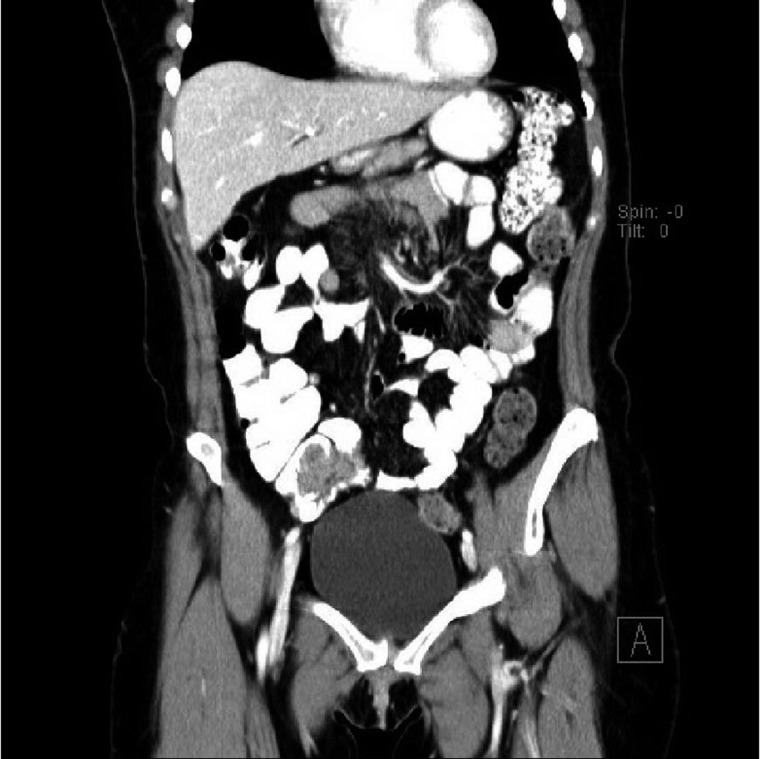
Contrast CT, coronal view of abdomen.
